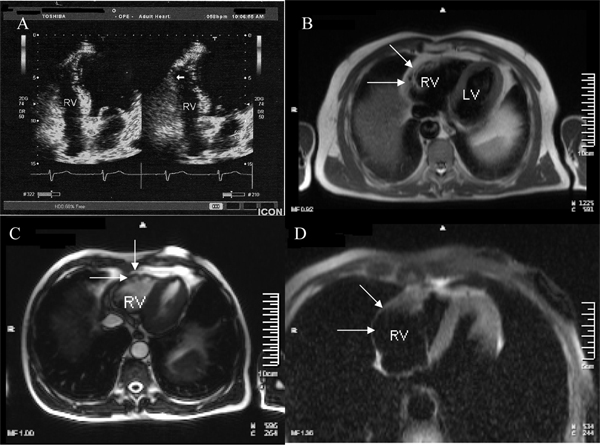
Panel (A) Cardiac echocardiogram (4 chamber view) shows slightly enlarged right ventricle with local dyskinesia and diastolic bulging of the free wall (arrow). Panel (B) Axial T1 weighted spin echo image shows diffuse hyperintense signal (arrows) in the free wall of the right ventricle. Panel (C) T2 weighted cine image with demonstration of multiple small aneurysms at the free wall of the right ventricle (arrows). Panel (D) Using an axial T1 weighted spin echo image with fat suppression, the hyperintense signal in the right ventricle wall was suppressed and identified as fatty tissue (arrows).
